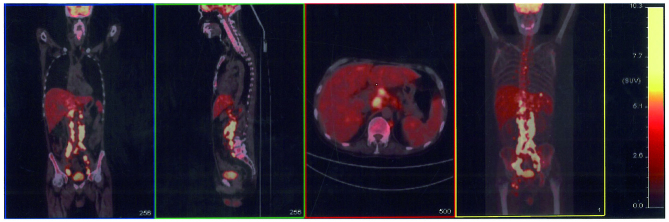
Positron emission tomography-computed tomography scan of the patient revealing multiple enlarged lymph nodes in the root of the left side of neck, mediastinum, bilateral hilus of the lungs, right cardiophrenic angle, hepatic portal area, peripancreatic area, diaphragm angle, posterior peritoneum, inter-mesangial area and anterior sacral area, and along the iliac blood vessels, bilateral iliac fossa, pelvic wall and bilateral lingual areas. The scans reveal multiple nodules in the bilateral lungs, an enlarged liver with nodular appearance of the parenchyma, an enlarged spleen with multiple low-density shadows in the parenchyma, and multiple regions of elevated fluorodeoxyglucose metabolism in the bones, such as the sternum, multiple vertebrae, and the bilateral ilia, pubis and ischia. A diagnosis of lymphoma was therefore considered.
